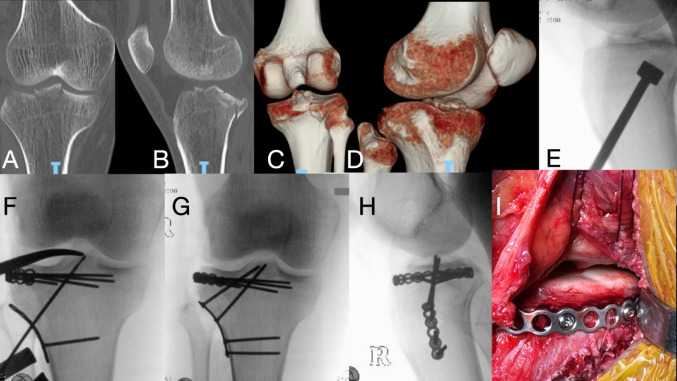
A, B Coronal and sagittal CT scans showing the tibial spine avulsion fracture associated with a posterolateral tibial plateau depression fracture. C, D Three-dimensional CT reconstructions demonstrating loss of the posterolateral tibial plateau rim. E Through an extended anterolateral approach, a cortical window was created in the lateral tibial plateau to allow insertion of a bone impactor, which was used to elevate and reduce the posterolateral depression. A 2.7-mm rim plate was applied through the anterolateral para-collateral fibular interval to contain the posterolateral rim, while rafting screws were used to support the reduced articular surface. A second 2.7-mm plate was placed to fix the cortical window and reduce the risk of a lateral tibial plateau cortical fracture. F Intraoperative fluoroscopic image demonstrating reduction of the tibial spine fragment using a Kelly clamp and the trajectory of the 2.0-mm drill directed toward the tibial spine for subsequent screw fixation. G, H Fluoroscopic images demonstrating anatomic reduction and final fixation. I Intraoperative photograph of the anterolateral approach demonstrating anatomic reduction of the articular surface
